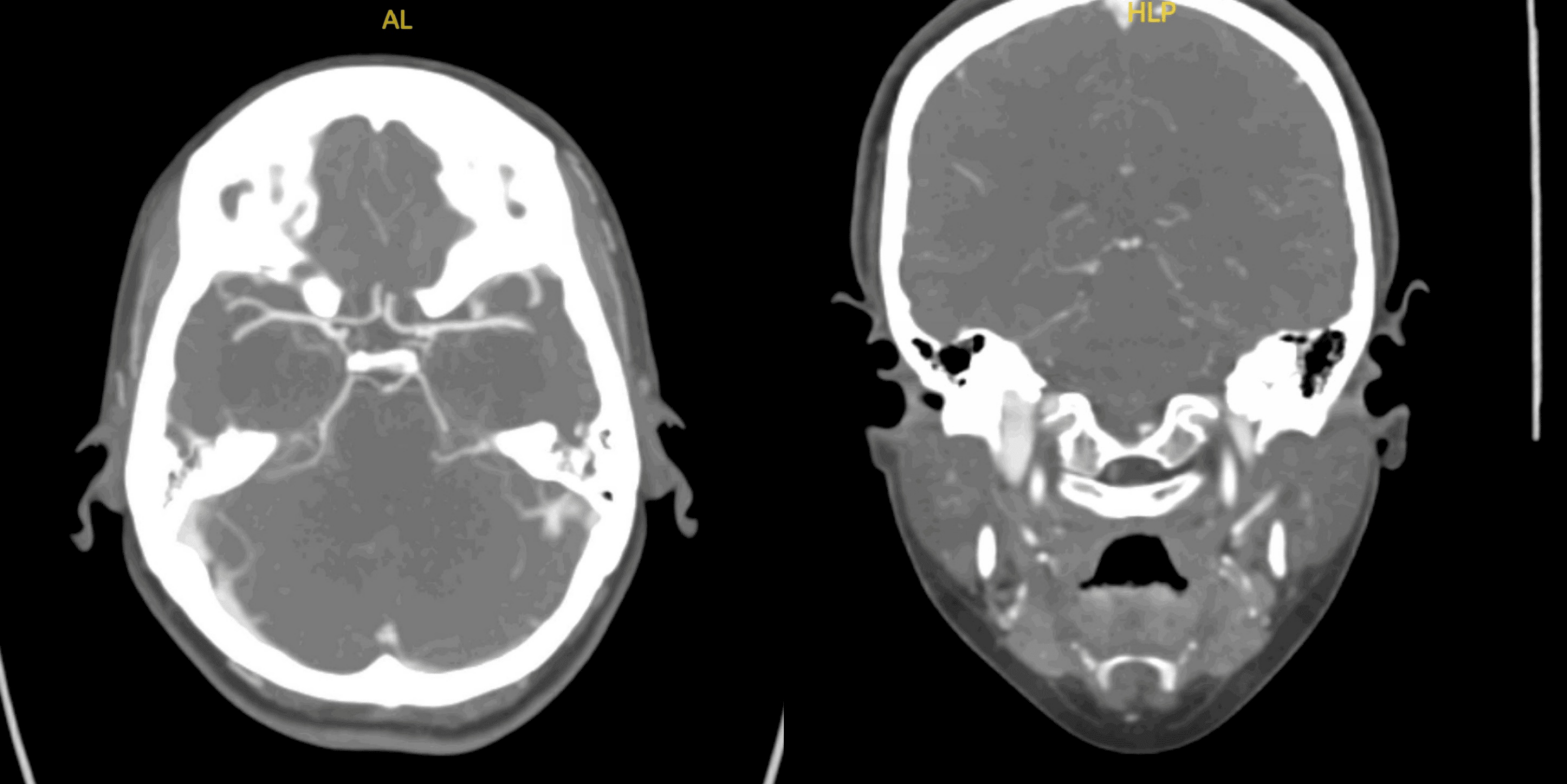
Essentially normal CT angiogram of the head and neck negative for high-grade stenosis, large vessel occlusion or aneurysm
